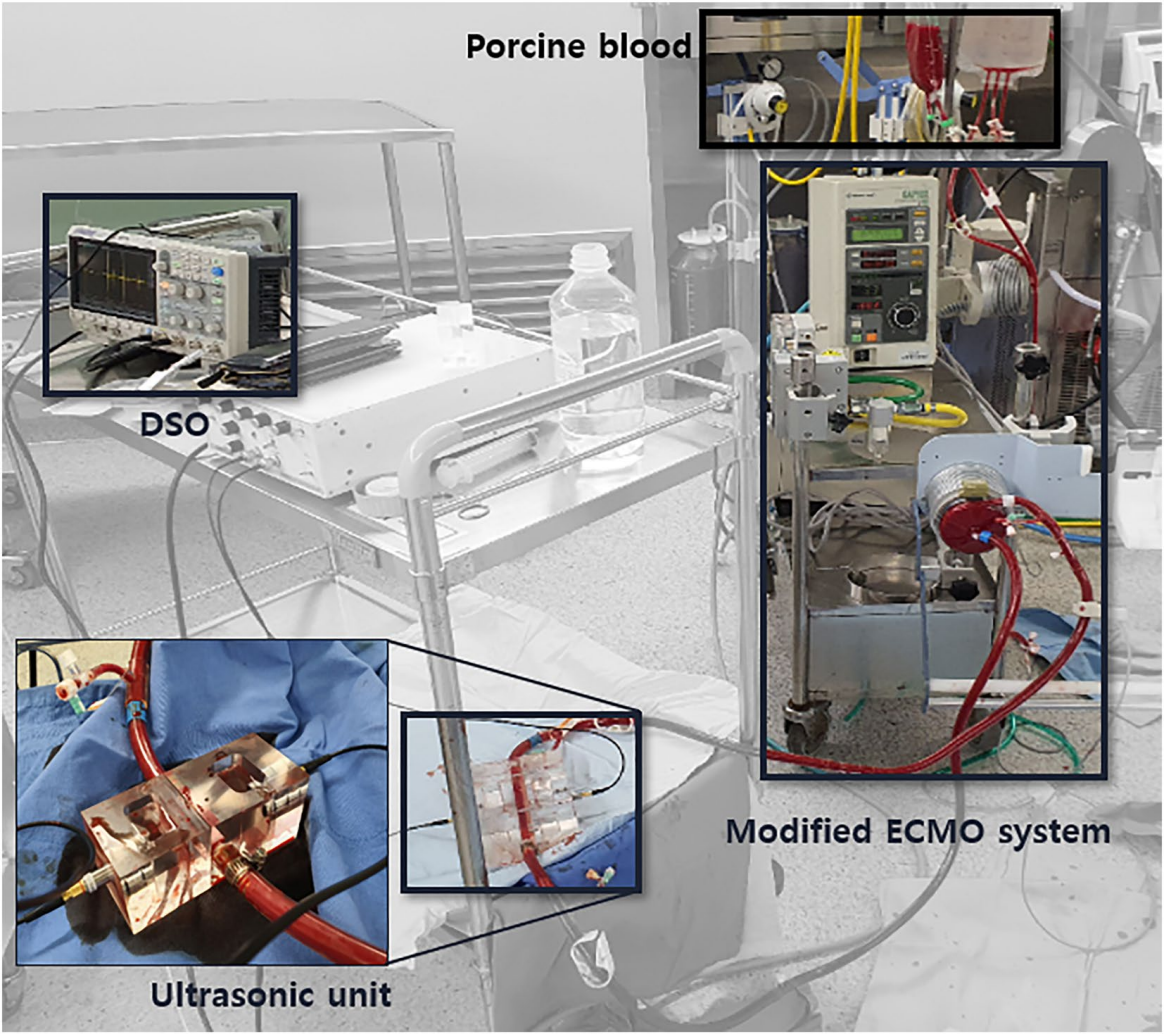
Real-time thrombus monitoring system with ultrasonic chambers. Modified ECMO system connected to ultrasonic sensors and a customized chamber. Magnified picture of the ultrasonic unit.
